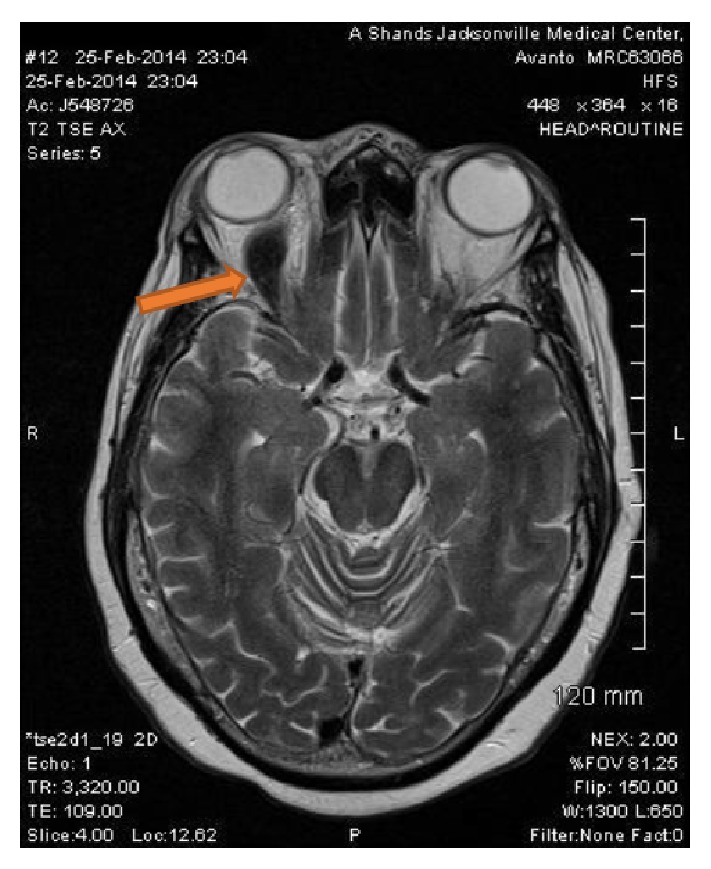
Brain MRI. Axial section (T2) at initial presentation. The arrow is pointing to decreased signal within right superior ophthalmic vein thrombosis.
